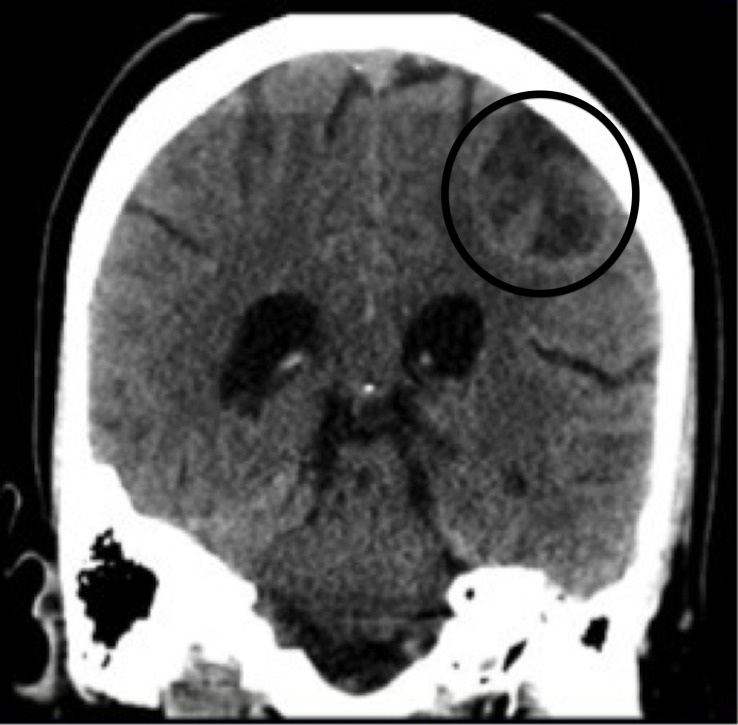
Computed tomography of brain with “open ring sign“ indicated by circle.
